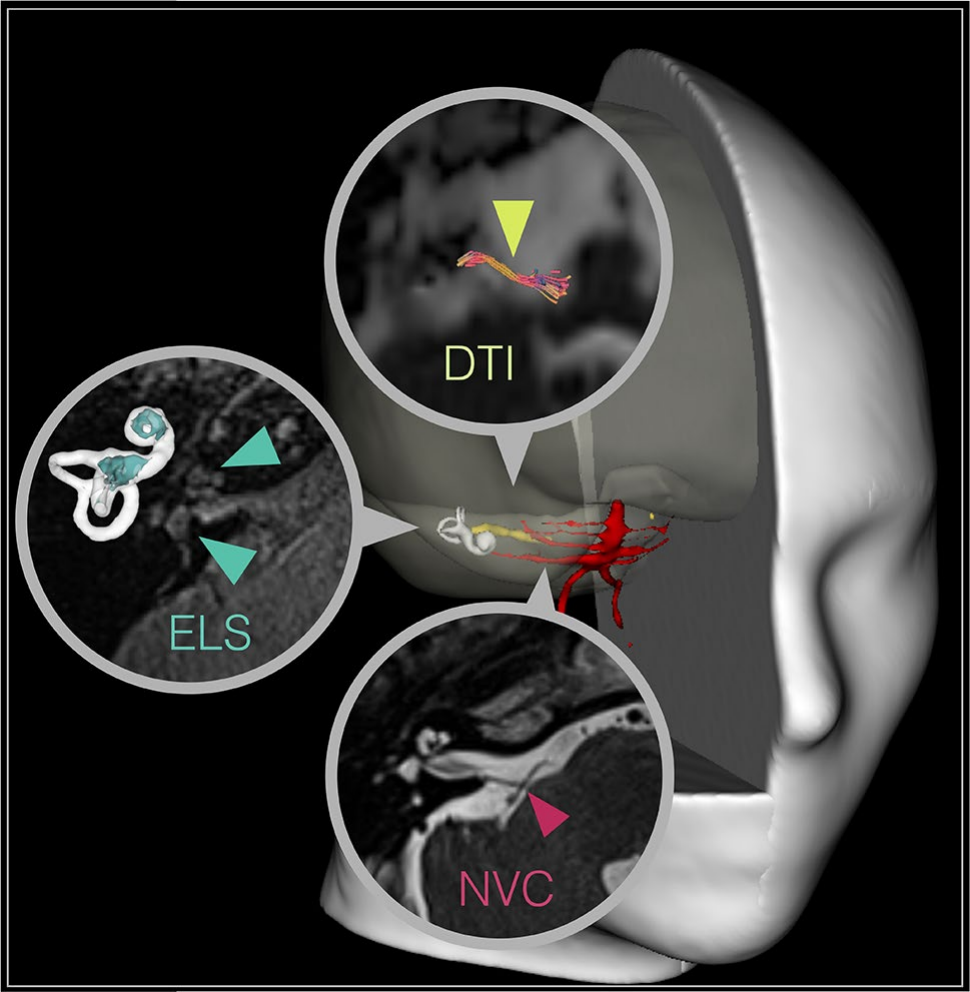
MR imaging approach tailored to VP. This study investigated the vestibular nerve with a MR imaging approach tailored to vestibular paroxysmia (VP). A 3D rendered T1-image visualizes the spatial relationship between overlaid blood vessels (red), the eighth cranial nerve (8 CN, yellow), and the vestibulocochlear end organ (white shell). These key structures are further shown magnified in a circle within a transversal slice of the cerebellopontine angle in the respective raw data with color-coded arrowheads pointing towards them. Particular emphasis was put on the site of neurovascular compression (NVC, behind red arrowhead in the lower circle), and the DTI tractography quantification of the cisternal segment of the eighth cranial nerve (behind yellow arrowhead in the upper circle) between the point of entry (PE: point of entry for afferent fibers, point of exit for efferent fibers into/out of the brainstem) and the internal auditory canal (IAC). In addition, the study focused on the volumetric quantification of the endolymphatic space (ELS, behind the turquoise arrowheads in the middle circle) within the membranous and bony labyrinth of the inner ear. The upper arrowhead points toward the cochlea, and the lower arrowhead points towards the vestibulum. The corresponding volumetric visualization of these structures (ELS in turquopoise) is depicted on the left, next to these structures. Visualization tools were a combination of 3D Slicer (https://www.slicer.org/, version 4.11 [36]) and DSI Studio (http://dsi-studio.labsolver.org/, version 2021-02-12).
